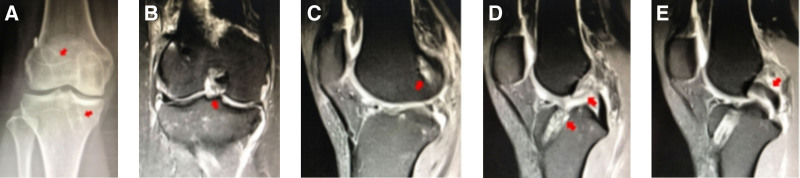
(A) DR: the tibial and femoral channels of the right knee were in good positions, albeit with slight enlargement of the bone tunnel. (B and C) MRI findings: the ACL was ruptured, and the signal of the tendon in the femoral tunnel was somewhat mixed when it was close to the joint cavity. (D and E) MRI findings: evidence of ACL rupture was observed, with the PCL demonstrating good morphology and tension; however, mixed signals were observed in the tendons within the tibial canal. ACL = anterior cruciate ligament, PCL = posterior cruciate ligament.
